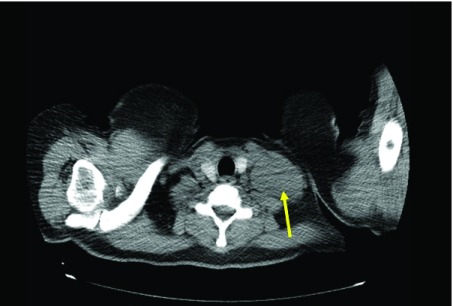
Chest CT scan. A soft tissue mass in the left supraclavicular region consistent with metastatic lymph node (yellow arrow).
